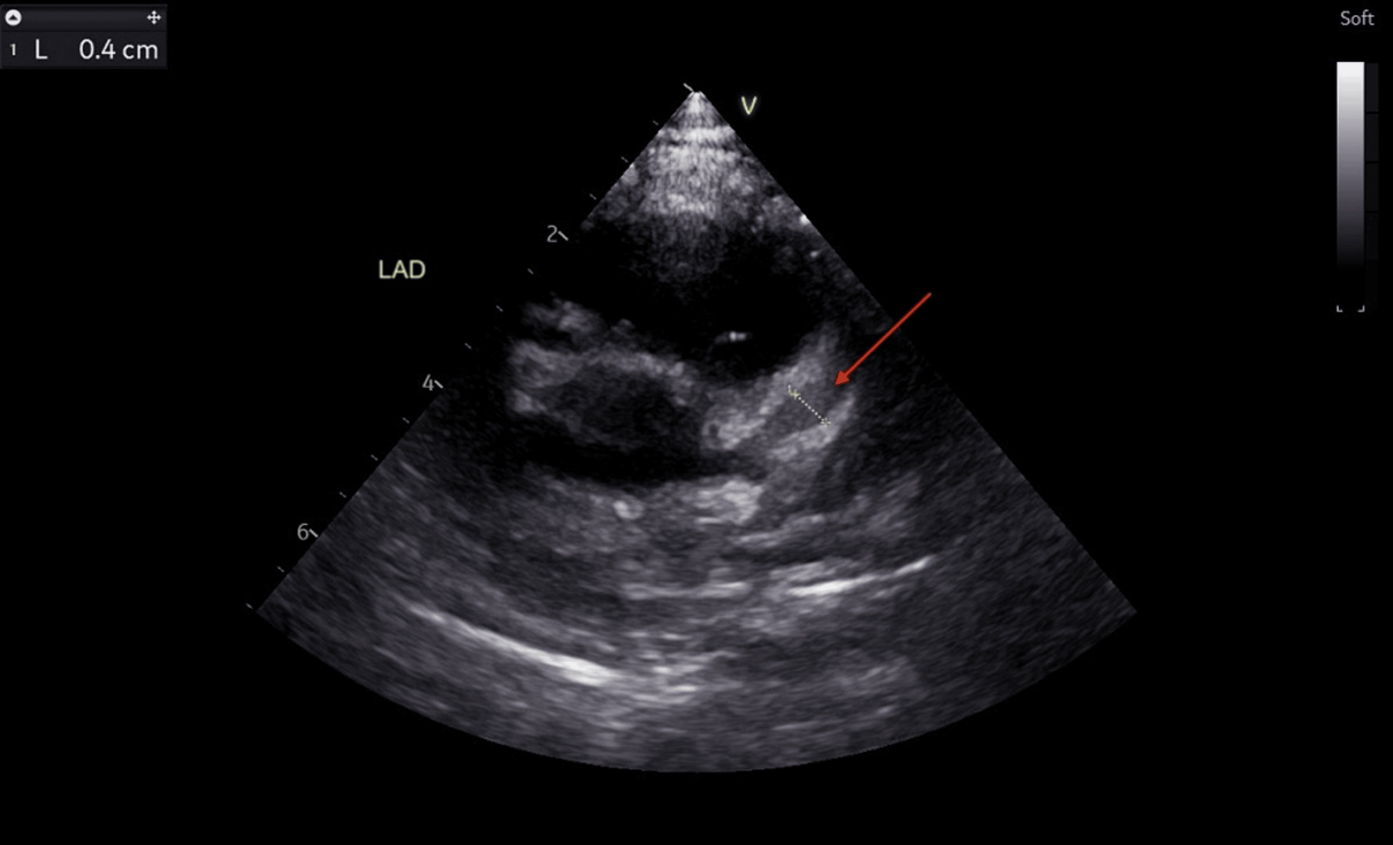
Echocardiogram showing a dilated left anterior descending artery. The red arrow points at the dilated left anterior descending artery measuring 4.0 mm (Z score: +6.72).
